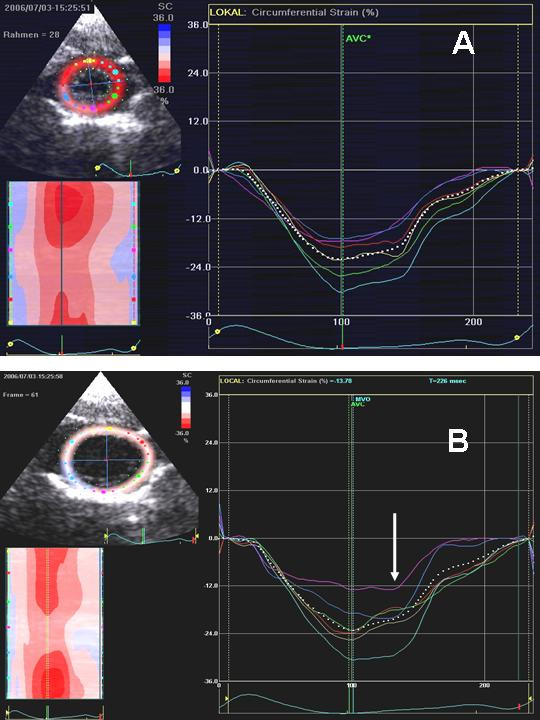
A and B Non-Doppler 2D strain measurements of the circumferential deformation of the anterior and inferior wall at baseline (A) and after treatment with reduction of the maximal strain und postsystolic shortening (arrow) in the inferior wall.
